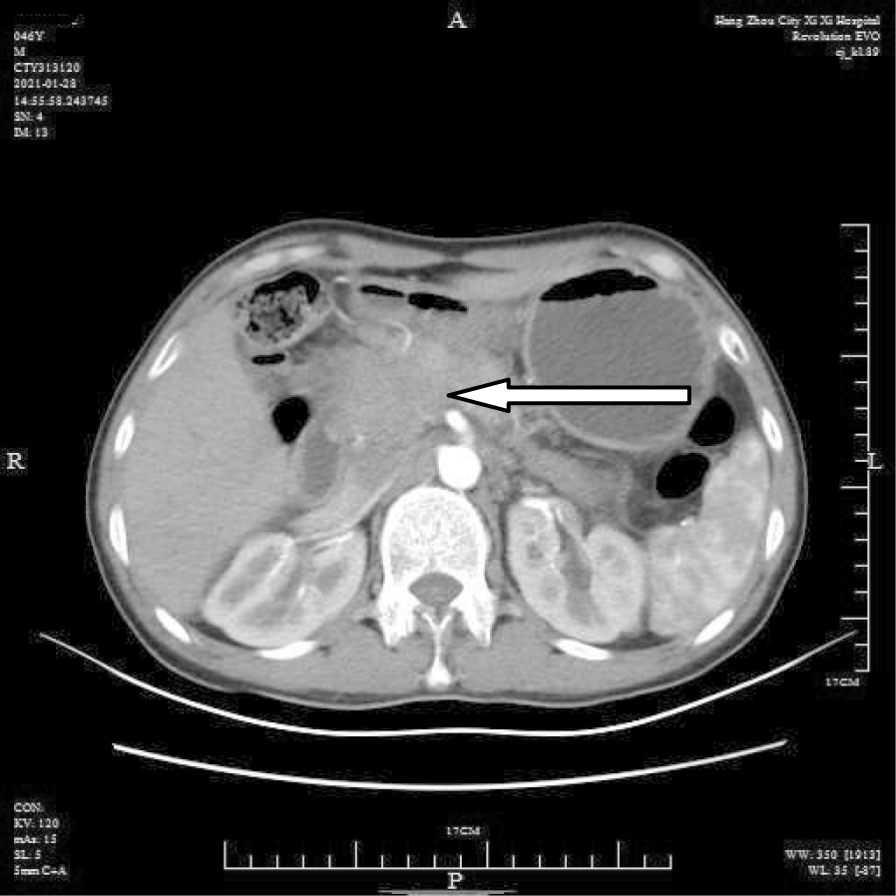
Head pancreas swollen in CT before the treatment
